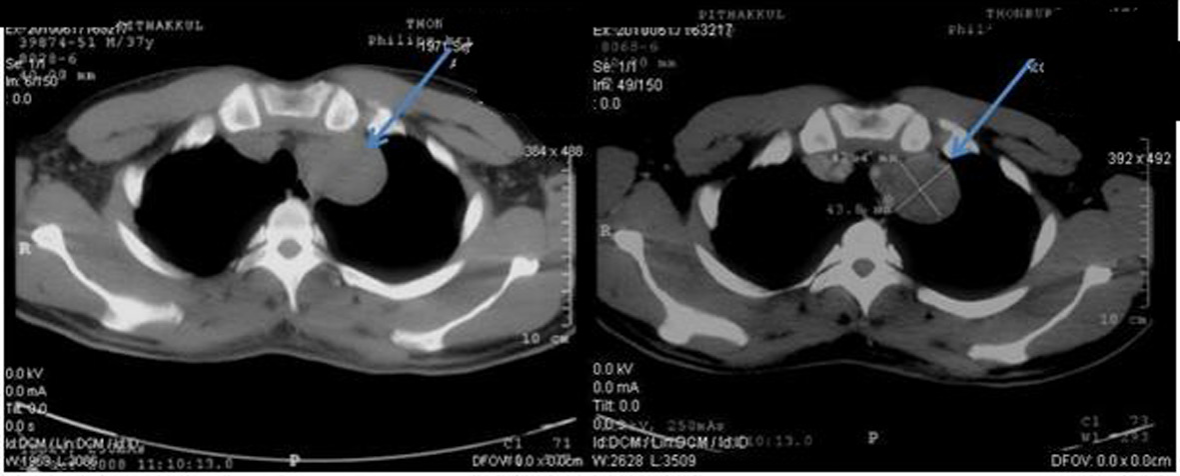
Pretreatment CT scan of chest in October 2008 (Pre-contrast on left side and post-contrast on right side) showing lobulated heterogeneous soft tissue density lesion at the apex of left lung.
